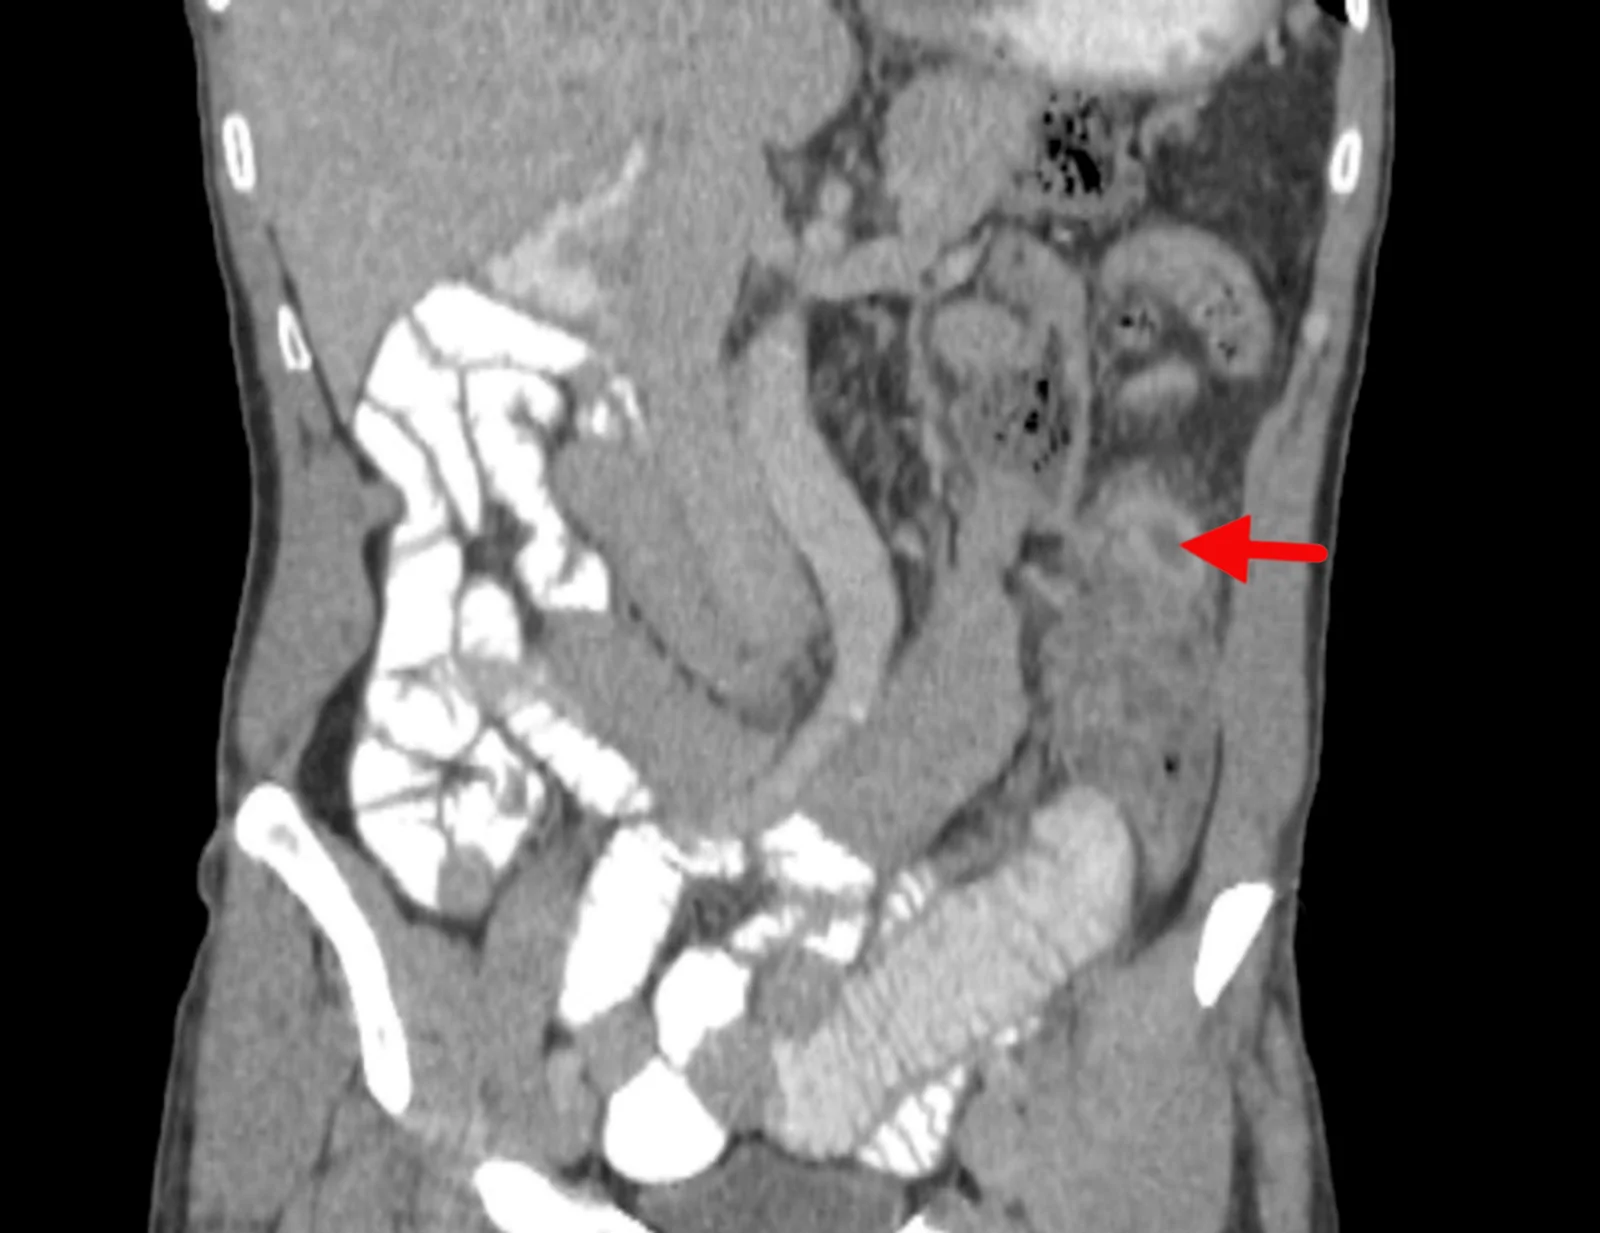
Left-sided appendicitis on coronal CT Coronal contrast-enhanced CT image demonstrating a thick-walled, dilated, inflamed appendix located in the left lower abdomen, consistent with left-sided acute appendicitis.
